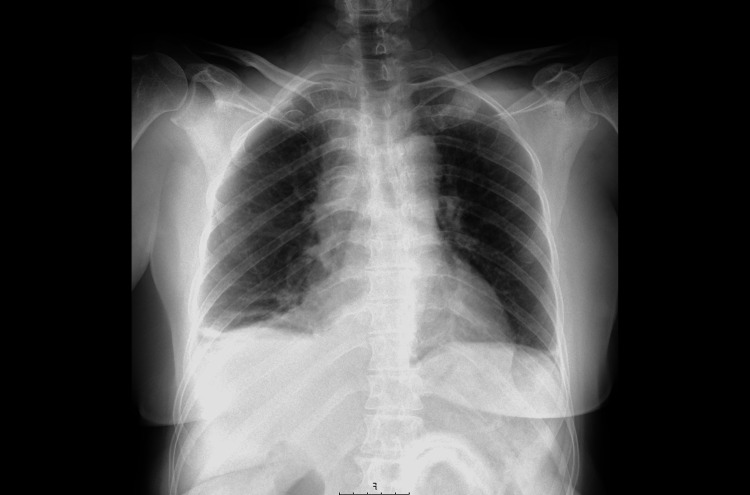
Thoracic X-ray at first ED admission Thoracic radiography with no evident lesions but with a very small pleural effusion on the right side
